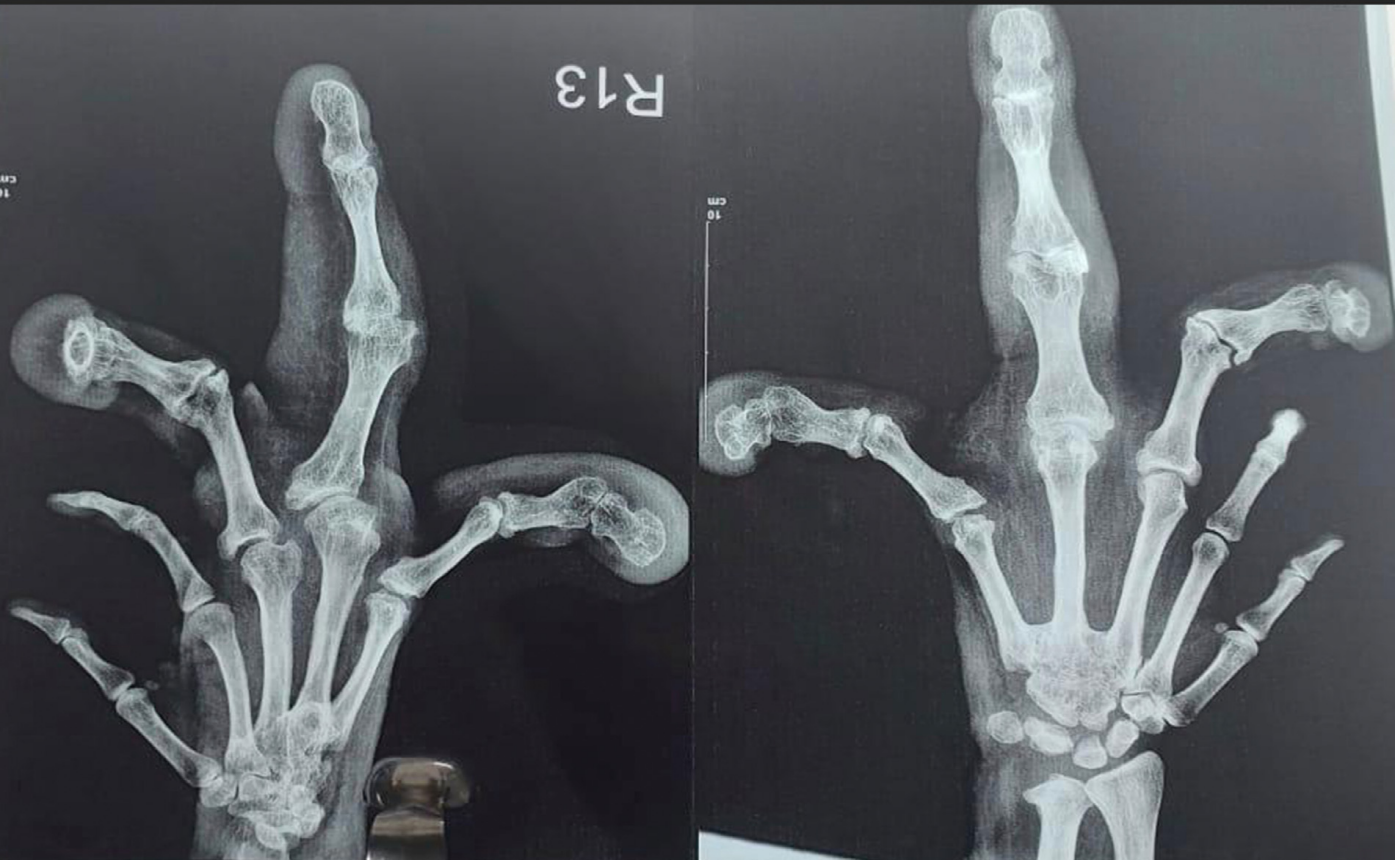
X-ray AP Oblique and PA view of Right hand showing abnormally enlarged third, fourth and fifth phalanges and metacarpals with hypertrophy of the soft tissues surrounding it.
